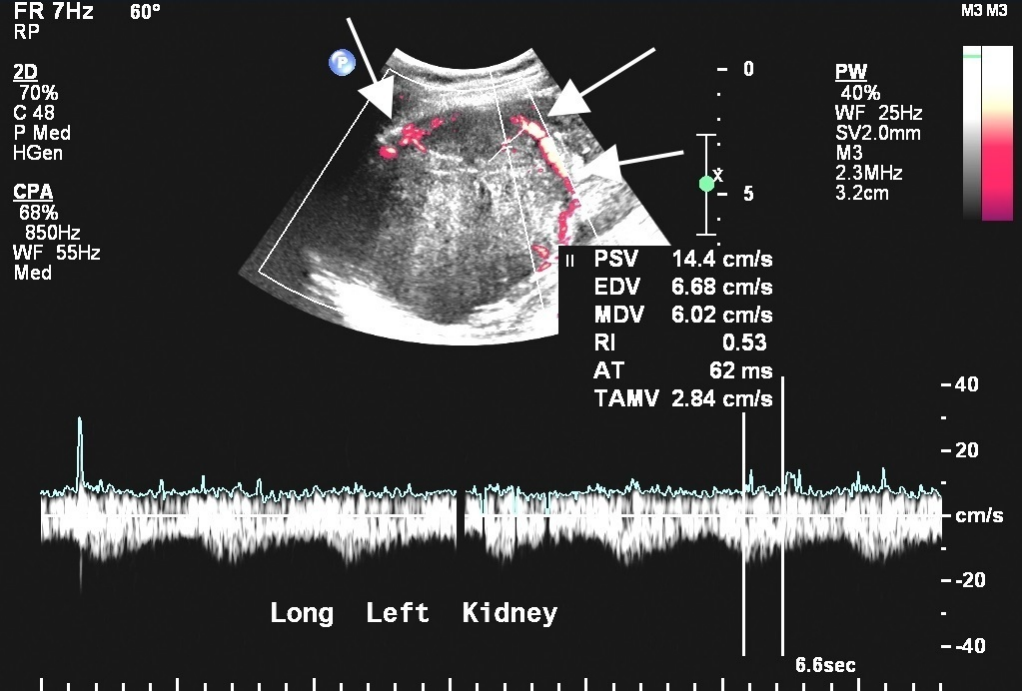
Ultrasound appearance of the SFT. A Doppler ultrasound image of the left kidney demonstrates a heterogeneous, predominantly hyperechoic left renal mass with peripheral color flow (white arrows).
